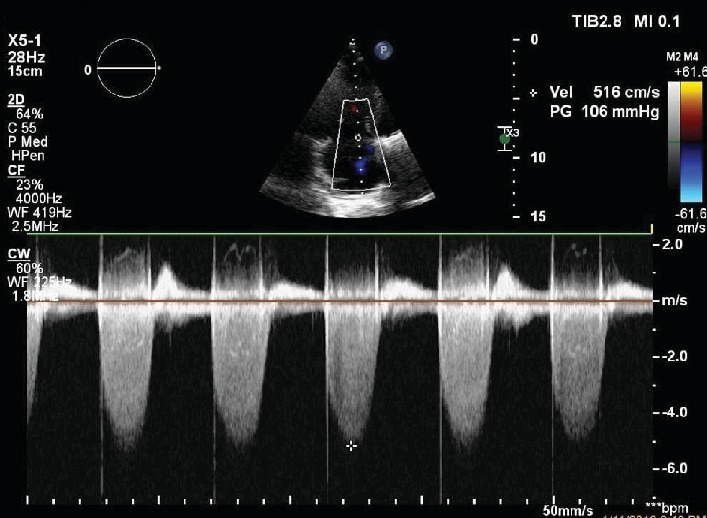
Continuous-wave Doppler interrogation of tricuspid regurgitation. Systolic gradient of 106 mmHg between the right ventricle and right atrium, revealing severe pulmonary hypertension.
